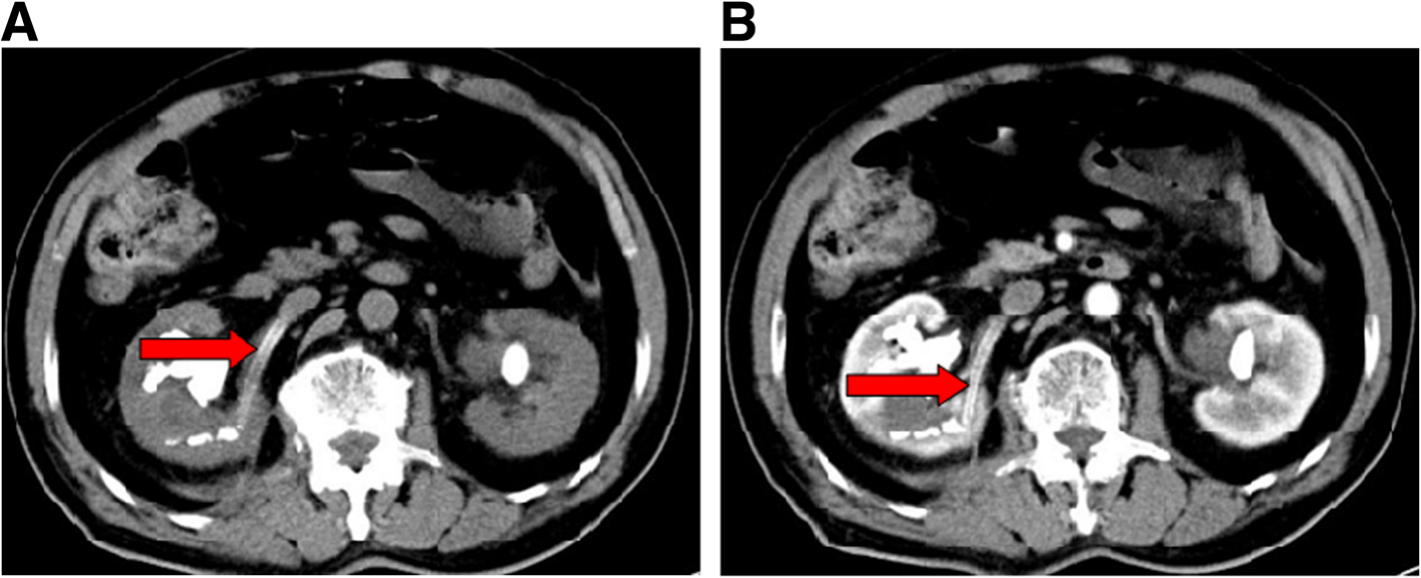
CT revealed the nephrostomy tube piercing into the right renal vein. a Plain of CT demonstrated nephrostomy catheter (*red arrow*) had traversed the lower pole of right kidney directly into the *right* renal vein. b Enhanced CT scan revealed that nephrostomy catheter (*red arrow*) had transversed *left* renal vein
